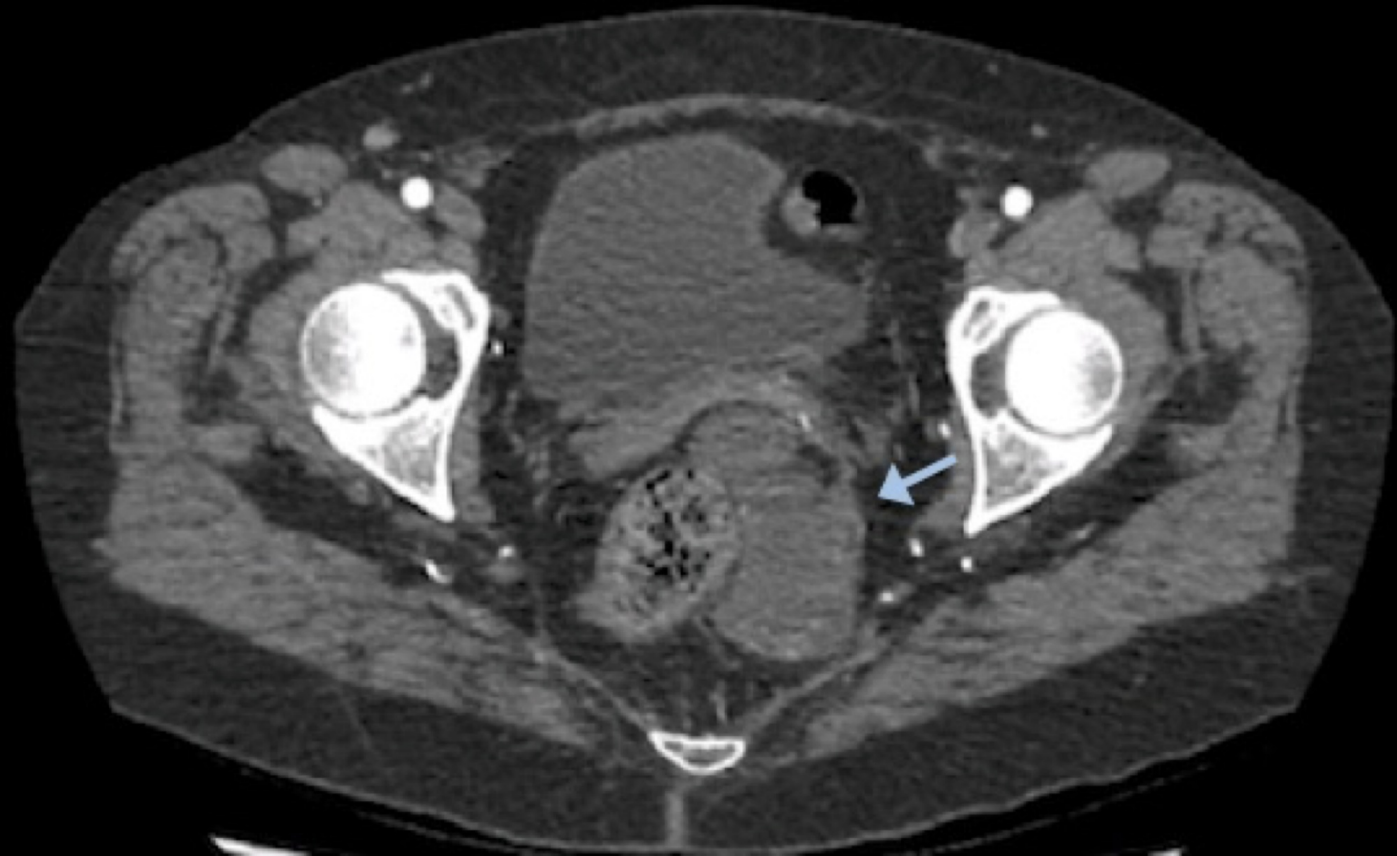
Abdominopelvic computed tomography (CT) scan revealing a left perirectal mass
